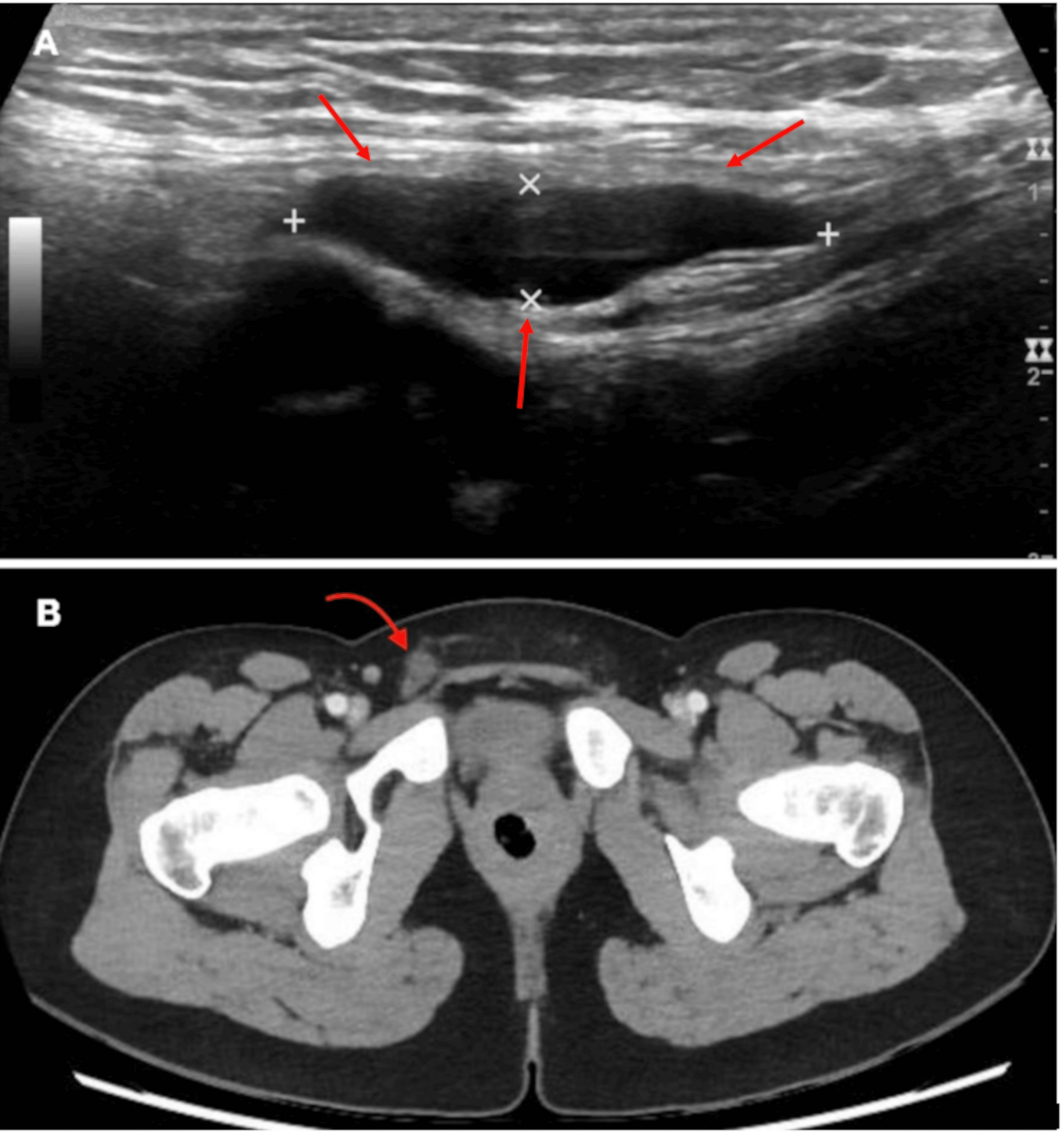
Patient imaging studies A) Ultrasound of the right inguinal region showing a cyst in the lower inguinal area measuring 2.8 x 0.6 cm, with no evidence of an abdominal wall defect. The image is consistent with a cyst of the right canal of Nuck. B) Abdominopelvic CT scan showing a rounded, fluid-filled lesion (9.6 Hounsfield units (HU)) in the right inguinal region with well-defined borders, homogeneous in appearance, measuring approximately 1 x 1.5 cm, and without communication with the peritoneal cavity (Type I). Red arrows indicate the imaging findings in each panel.
